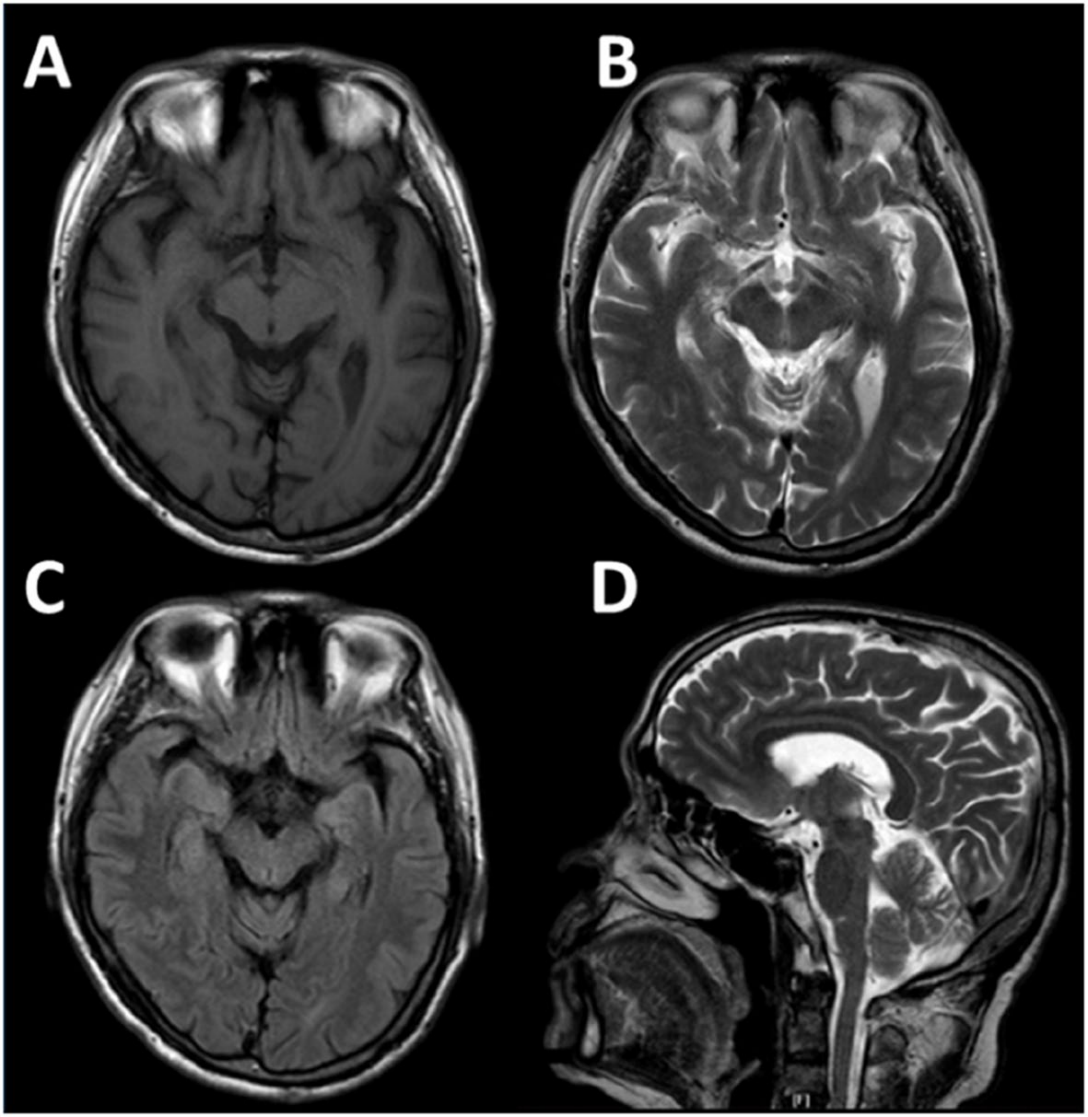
Brain structure of PD patients with magnetic resonance imaging. The MRI imaging performed transverse scans of T1-weighted (A), T2-weighted (B), T2-weighted (C), and sagittal scans of T2-weighted (D) images in 2017. No severe morphological changes in the brain were reported
